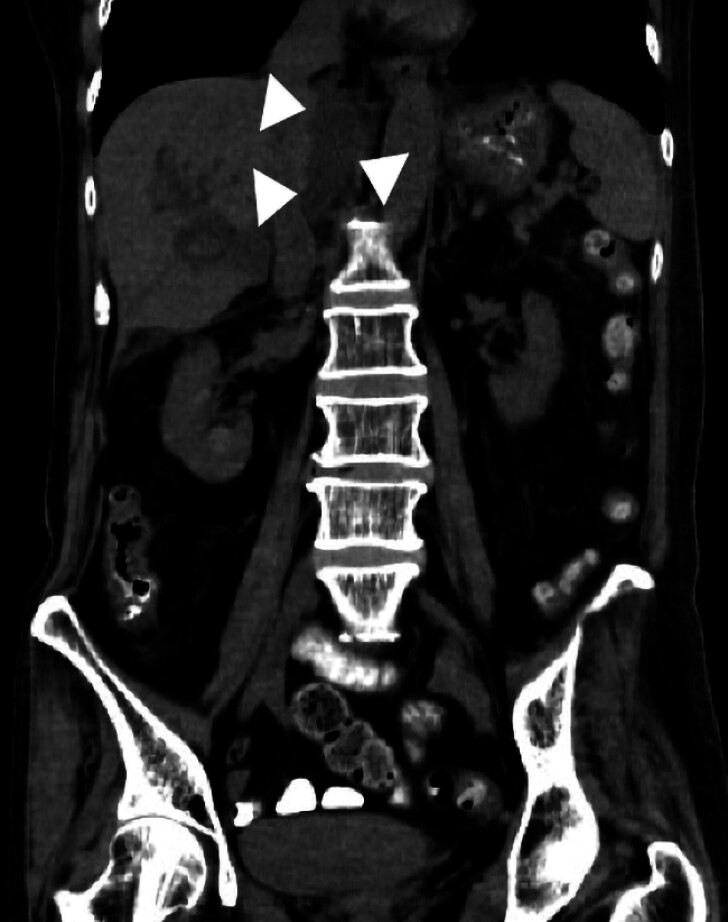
Computed tomography image showing biliary leakage around the liver on day 1 after the endoscopic ultrasonography-guided hepaticogastrostomy procedure.
